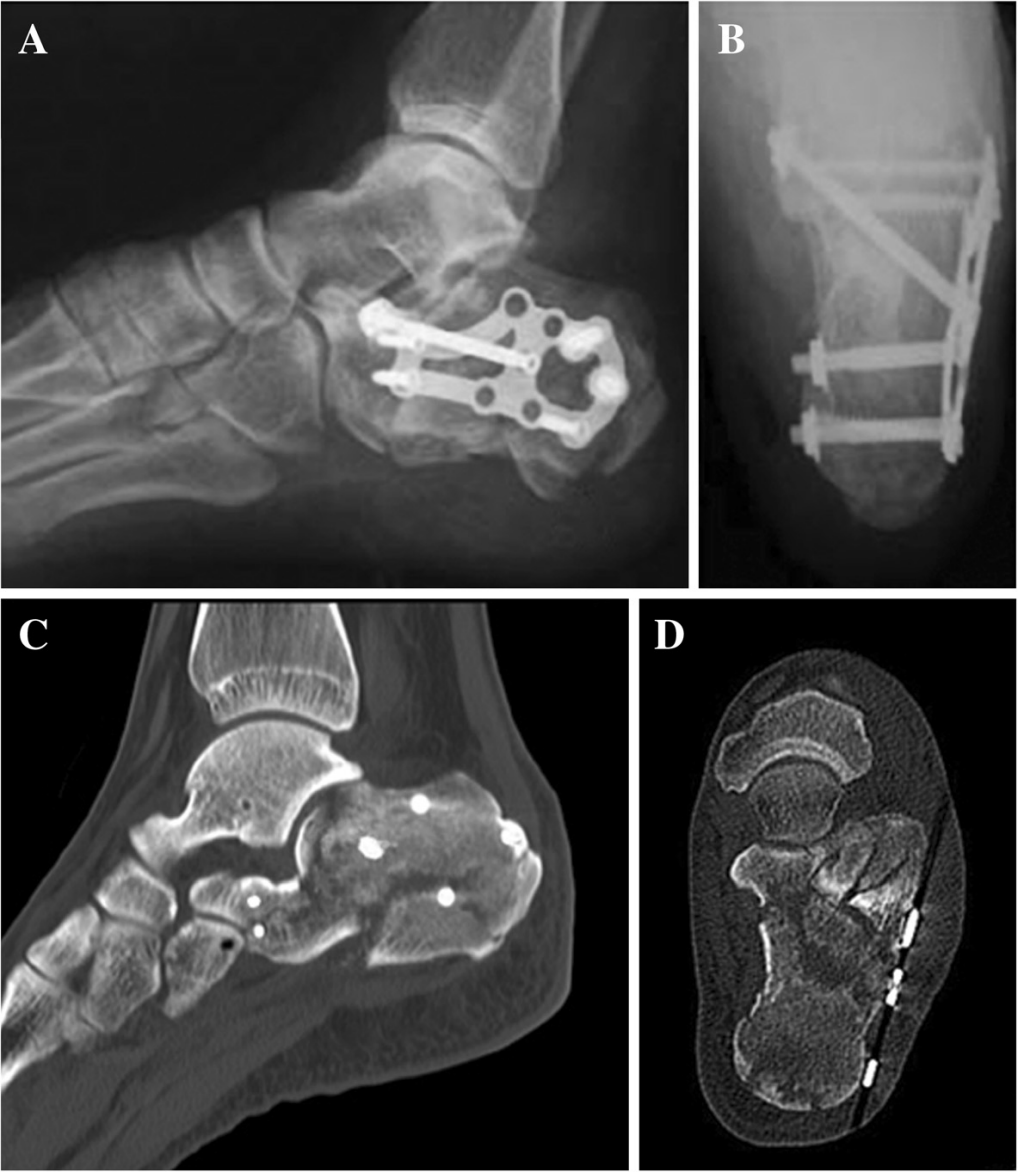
Postoperative radiographs (A, lateral view; B, axial view) and CT images (C, the sagittal image; D, the coronal image) of the same patient show that the gross anatomy of the calcaneus was restored and that the subtalar joint was significantly reduced.
